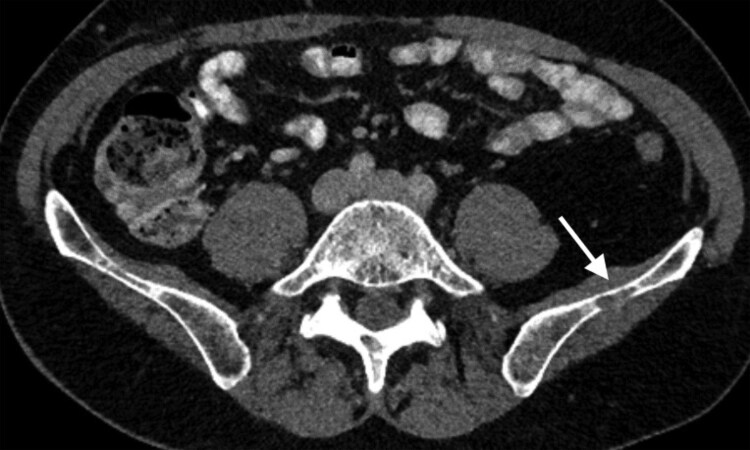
CT scan imaging demonstrates a 5 × 2 mm osteolytic lesion in the left iliac bone with cortical destruction (white arrow).
